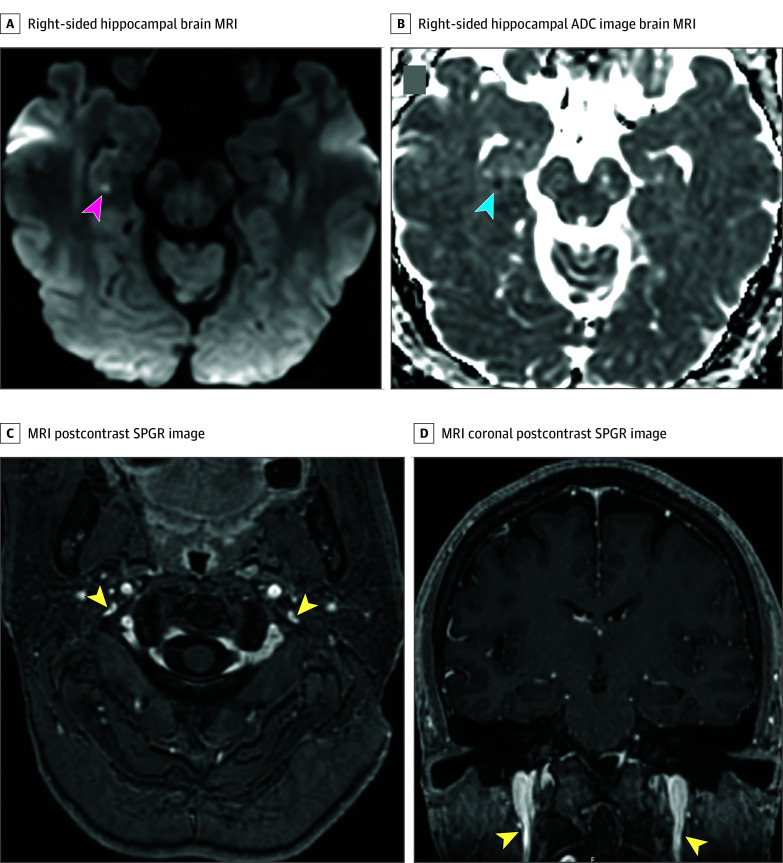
Brain Magnetic Resonance Imaging (MRI) Appearances and the Corresponding Severe Styloidogenic Jugular Venous Compression (SJVC) Stenosis of the Internal Jugular Veins (IJVs) in the Upper Neck of a 61-Year-Old Female With Clinical Transient Global Amnesia (TGA) A, Focal punctate right-sided hippocampal hyperintensity (pink arrow) of axial MRI-diffusion–weighted imaging associated with restricted diffusion. The patient was imaged 24 h after TGA onset. B, The corresponding axial apparent diffusion coefficient (ADC) image shows hypointensity (blue arrow) of the same lesion. C, The MRI axial postcontrast spoiled gradient echo (SPGR) image shows that the patient had severe narrowing of bilateral IJVs (yellow arrows) associated with SJVC. D, A corresponding coronal postcontrast SPGR image shows the same severe SJVC narrowing of bilateral IJVs (yellow arrows).
